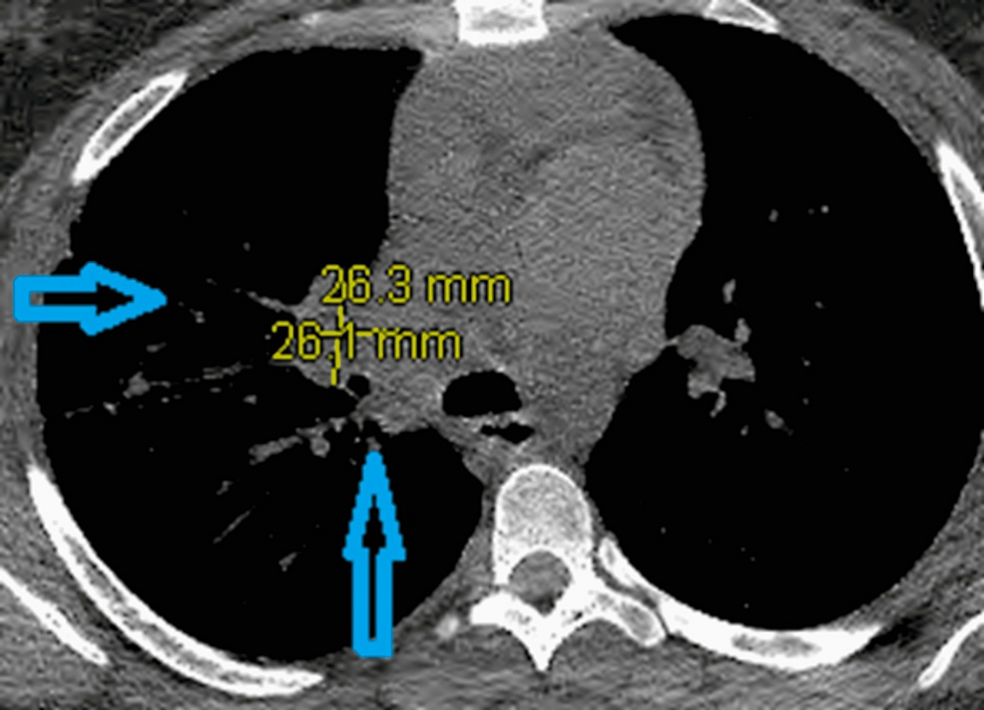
CT chest (axial image) revealed interval reduction of the right hilar mass (vertical blue arrow) with decreased RUL opacities in comparison to Figure 2A (horizontal blue arrow) RUL: right upper lobe
